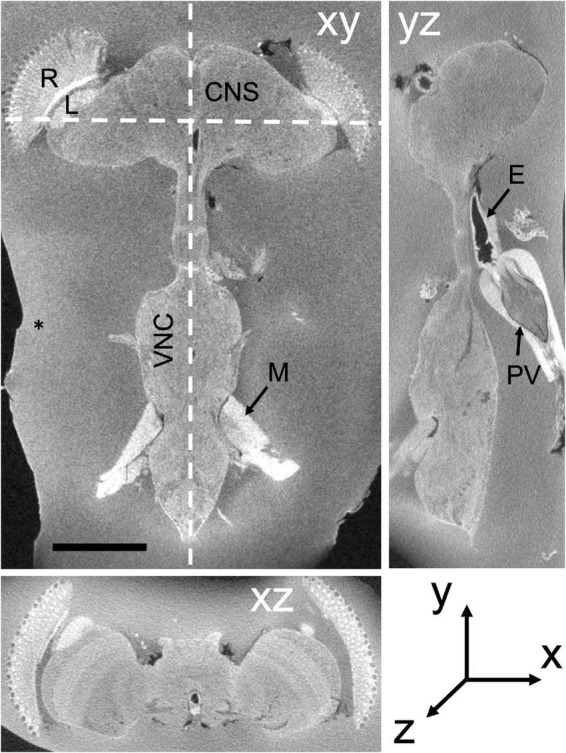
Soft X-ray dissected *Drosophila* CNS in three orthogonal planes, xy, yz, and xz. xy: frontal slice of the entire CNS, including the VNC. yz sagittal plane. xz transverse plane. Different zones in the surrounding BSA reveal successive additions of the cross-linked protein. E, esophagus; L, lamina; M, muscle; R, retina; PV, proventriculus. Scale bar in xy: 200 μm.
